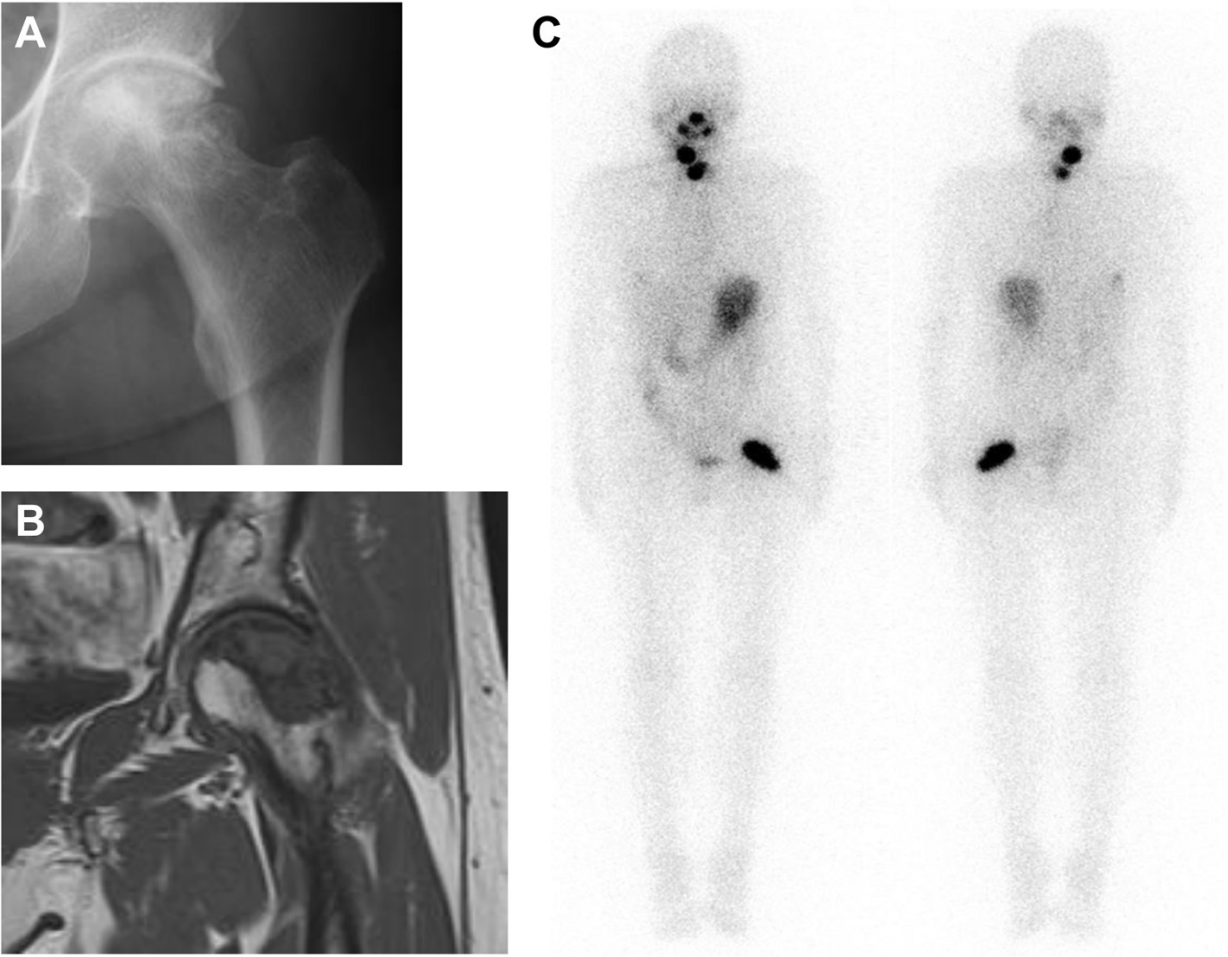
a X-ray of the hip joint 3 years after initial consultation, showing that the collapsed area had expanded. b Coronal T1 weighted MR image showing expanded low intensity of the left femoral head lesion. c
